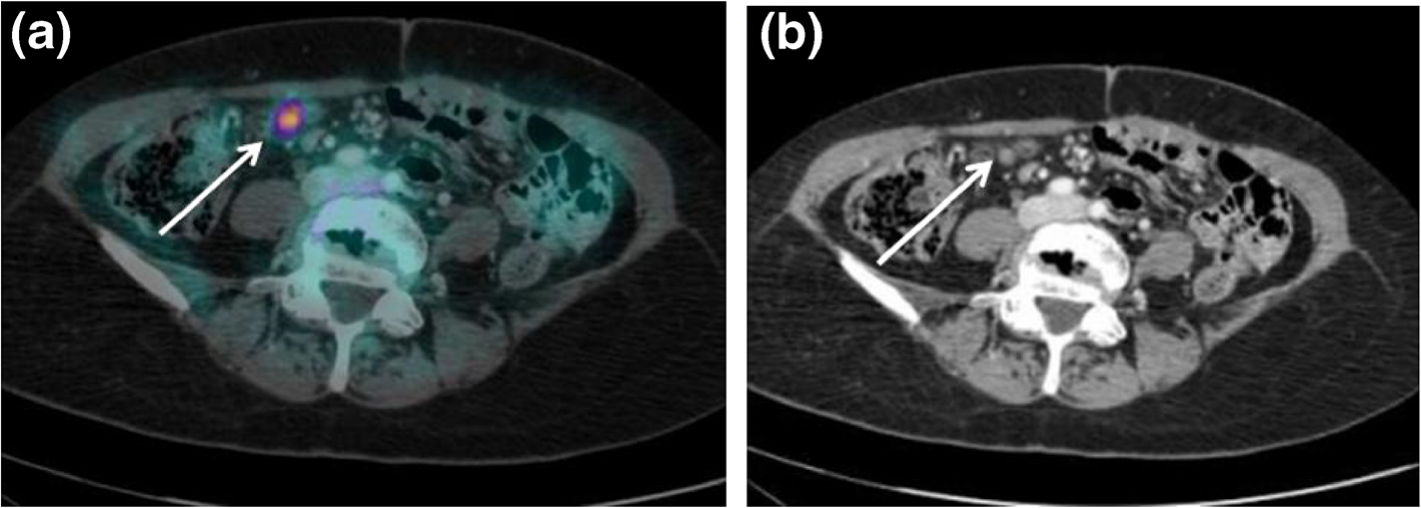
A 54-year-old female patient previously submitted for surgical treatment for primary colon cancer and with a known, single liver metastasis. This patient underwent contrast-enhanced PET/CT for the exclusion of other metastatic lesions, as she was a candidate for surgical resection of the hepatic localization. (a) Axial, contrast-enhanced, full-dose CT shows a suspicious 8-mm solid lesion close to the small bowel. (b) The lesion is highly FDG-avid on contrast-enhanced PET/CT. However, the pathologic nature of this finding is clearly evident only on contrast-enhanced CT, whereas using standard CT, it could have been deemed as unspecific bowel FDG-activity. This patient thus avoided inappropriate surgical treatment and was referred for chemotherapy. PET, positron emission tomography; CT, computed tomography; FDG, [^18^ F] fluorodeoxyglucose.
